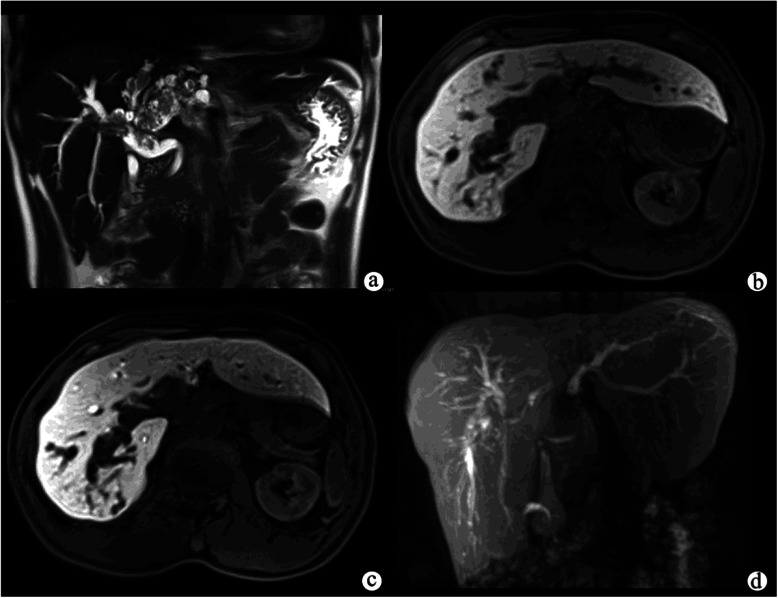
Papillary cystadenocarcinomain a patient with right abdominal pain. A T2-weighted image (a) showed marked dilatation of the intrahepatic and extrahepatic bile ducts with multiple heterogeneously hypointense polypoid nodules on the surface. The hepatocellular phase (b) showed that the polypoid nodules were homogeneously hypointense, and nearly no Gd-EOB-DTPA was seen in the dilated bile duct. The biliary phase (c) showed that most of the dilated bile duct was filled with Gd-EOB-DTPA, indicating that the function of biliary system was normal. Gd-EOB-DTPA-enhanced MR cholangiography (d) showed that the intrahepatic and extrahepatic bile ducts were well-filled with contrast agent.
